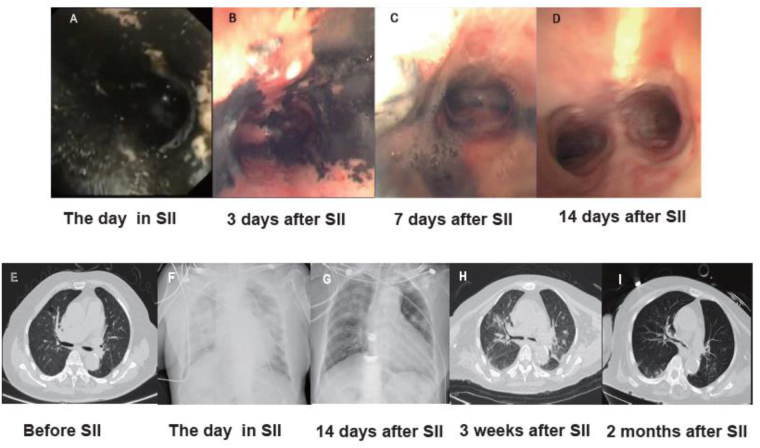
Bronchoscopic image of bronchial tube and pulmonary imaging. (A) Bronchoscopy revealed abundant black soot and serious injury of bronchial tube in the day of inhalation injury. (B) Bronchoscopy showed still seriously injured bronchial tube of patient after 3 days of inhalation injury. (C) Hyper mucus secretion was observed in bronchoscopy after 7 days of inhalation injury (D) Mucus had disappeared and wound surface of bronchial inhalation injury recovered basically including reduced mucosal edema and hemorrhage, after 10 days treatment of mesenchymal stem cell exosome nebulization. (E) Chest computed tomography showed the pulmonary condition of patient in 5 months before inhalation injury. (F) Chest X-ray revealed the severe inhalation injury in the day of inhalation injury. (G) Chest X-ray on the tenth days after inhalation injury revealed effective treatment of mesenchymal stem cell exosome nebulization. (H) Chest computed tomography showed recovery of lung in the day after 3 weeks of inhalation injury. (I) Chest computed tomography in 2 months’ follow-up revealed good recovery of pulmonary parenchyma and no severe pulmonary fibrosis. Abbreviation: SII, severe inhalation injury.
